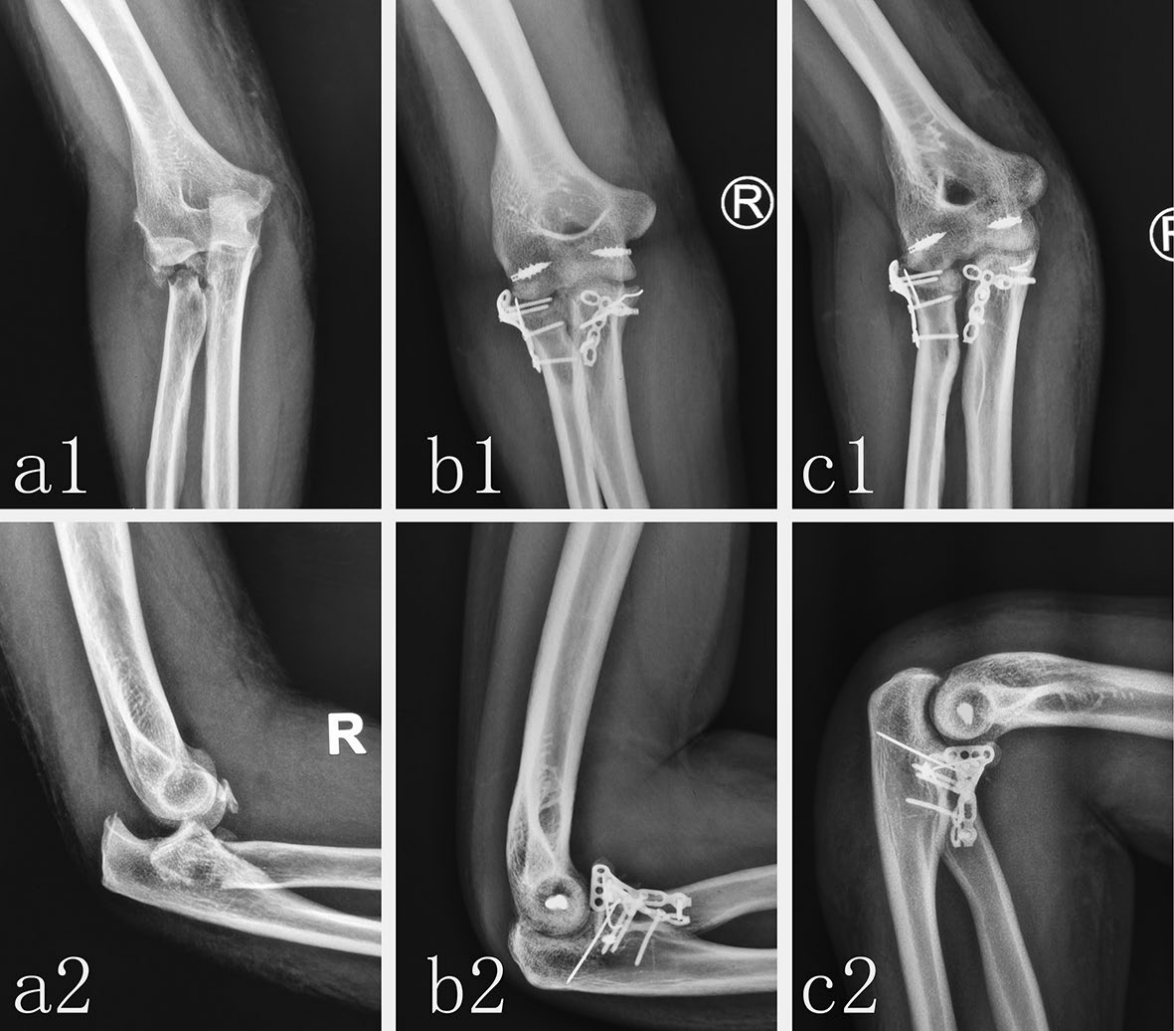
Radiological image of typical patient with TTE using ORIF. The preoperative anteroposterior X-ray (a1) and lateral X-ray (a2) showed the posterior dislocation of right elbow joint, the fracture of radial head and coronoid process. An anteroposterior X-ray (b1) and lateral X-ray (b2) at postoperative follow-up showed that the fracture of coronoid process and radial head were fixed by miniplate and the LCL and MCL of elbow were repaired by suture anchor. An anteroposterior X-ray (c1) and lateral X-ray (c2) at 6-months postoperative follow-up showed that fracture had healed well and the dislocation and instability of elbow were not observed.
